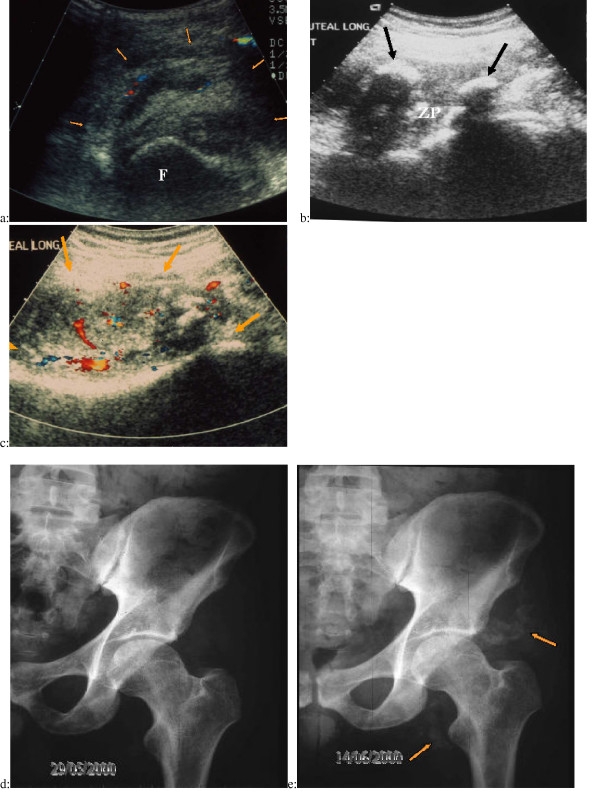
Ultrasound and color Doppler examination and digital radiographs of suspected NPOA a: Axial US view combined with color Doppler of the anterior side of the left hip in a paraplegic patient presenting acute limitation and inflammation of this joint. The striation of the psoas iliaque muscle, normally detectable at the anterior part of the hip joint with US examination, has disappeared. A relatively well defined mass (orange arrows) is detectable at the anterior part of the left femoral head (F). This mass is very heterogeneous with mixed hypo and hyper echoic areas. Color Doppler enables visualization of vessels in the mass (red and blue Doppler signals). A mass effect is visible on the femoral vessels (top right of the view). b: Same patient, one week later, axial US view of the posterior side of the left hip. The classical zone phenomenon (ZP) is detectable with a central hypoechoic area surrounded by hyper echoic nodules with posterior attenuation(black arrows). c: Axial US examination at the same day combined with color Doppler view of the posterior side of the left hip. A posterior mass (orange arrows) is also visible in the gluteal muscles, very heterogeneous with mixed hypo and hyper echoic areas. Color Doppler reveals large vessels in the mass (red and blue Doppler signals). d: Plain radiographs of the left hip obtained the same day as first US examination: Any sign of ossification is visible while a well defined mass is detected by US examination. e: Plain radiographs of the left hip obtained two weeks after: Early anterior and posterior NPOA ossification is only slightly visible two weeks (Orange arrows) after the initial clinical signs whereas the US examination was initially positive.
